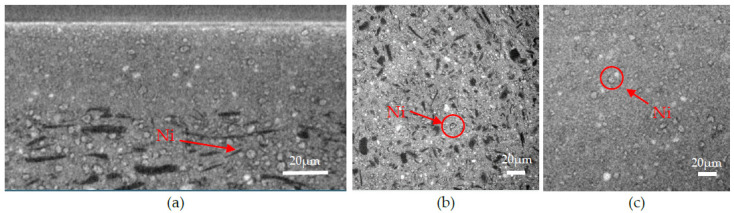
X-ray tomography images of the reduced sample: (a) cross-section along the *X*-axis; (b) cross-section of the anode along the *Y*-axis; (c) cross-section of the AFL along the *Y*-axis.
